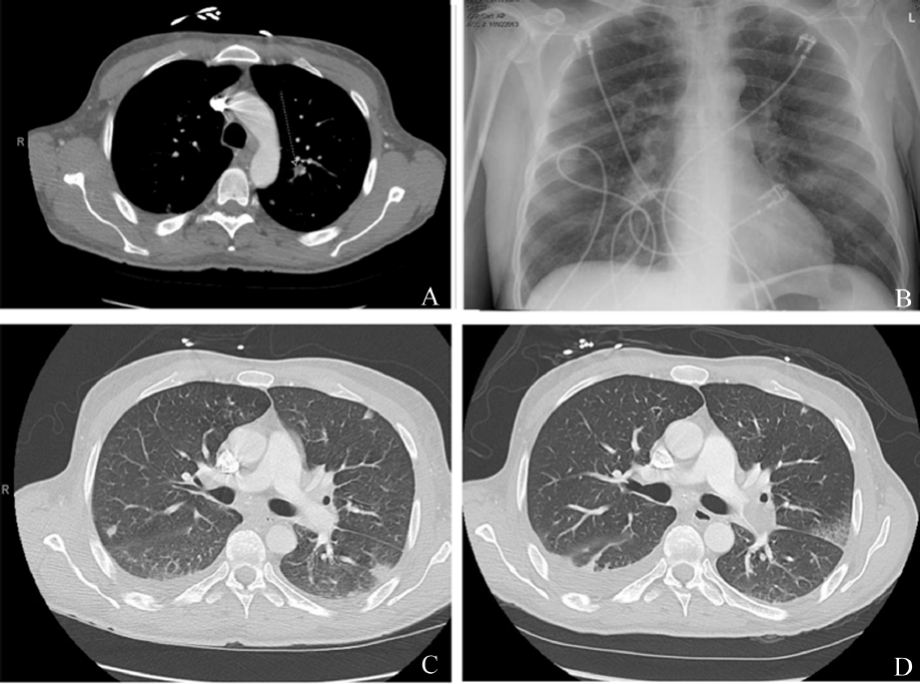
(A) Septic emboli on CT of the chest on the left middle lobe. (B) Septic emboli subtle on the left lung. (C) Initial CT PE protocol negative for PE. (D) Repeat CT PE protocol positive for large left-sided PE.
